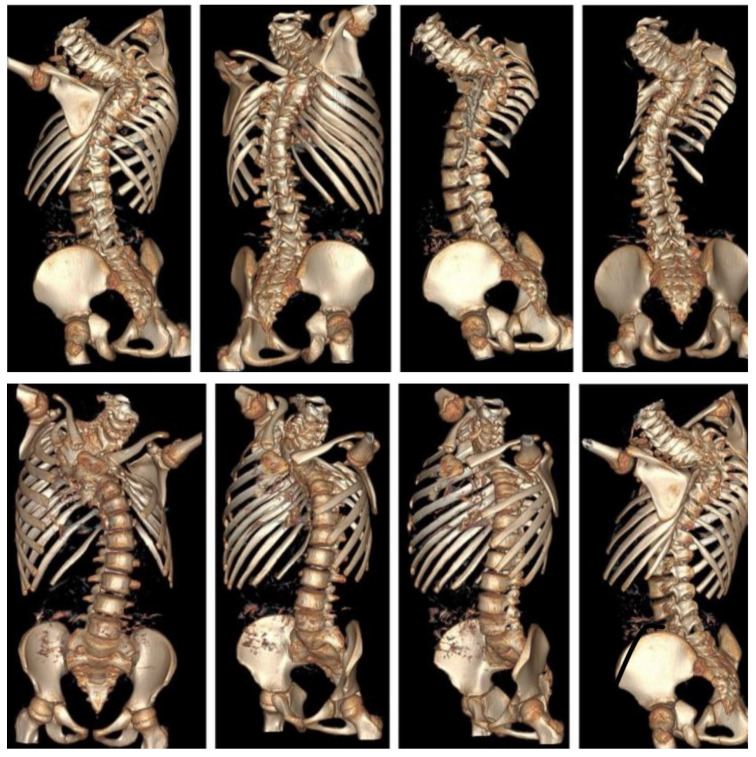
3D CT reconstruction of 5-year-old girl with congenital progressive and rigid kyphoscoliosis treated with posterior vertebral column resection at T3–T4 level.
